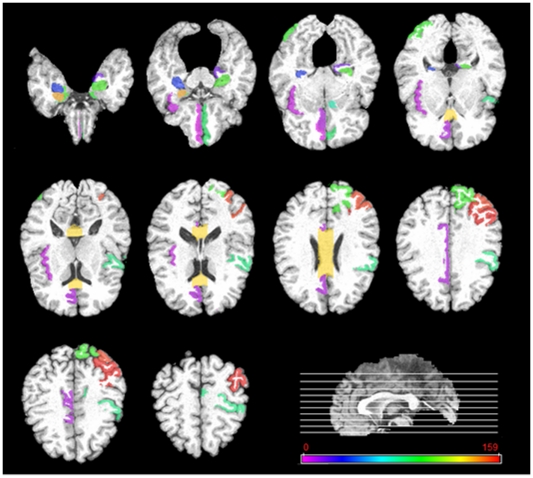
Top 20% brain regions detected by the longitudinal feature selection method on MRI images.
